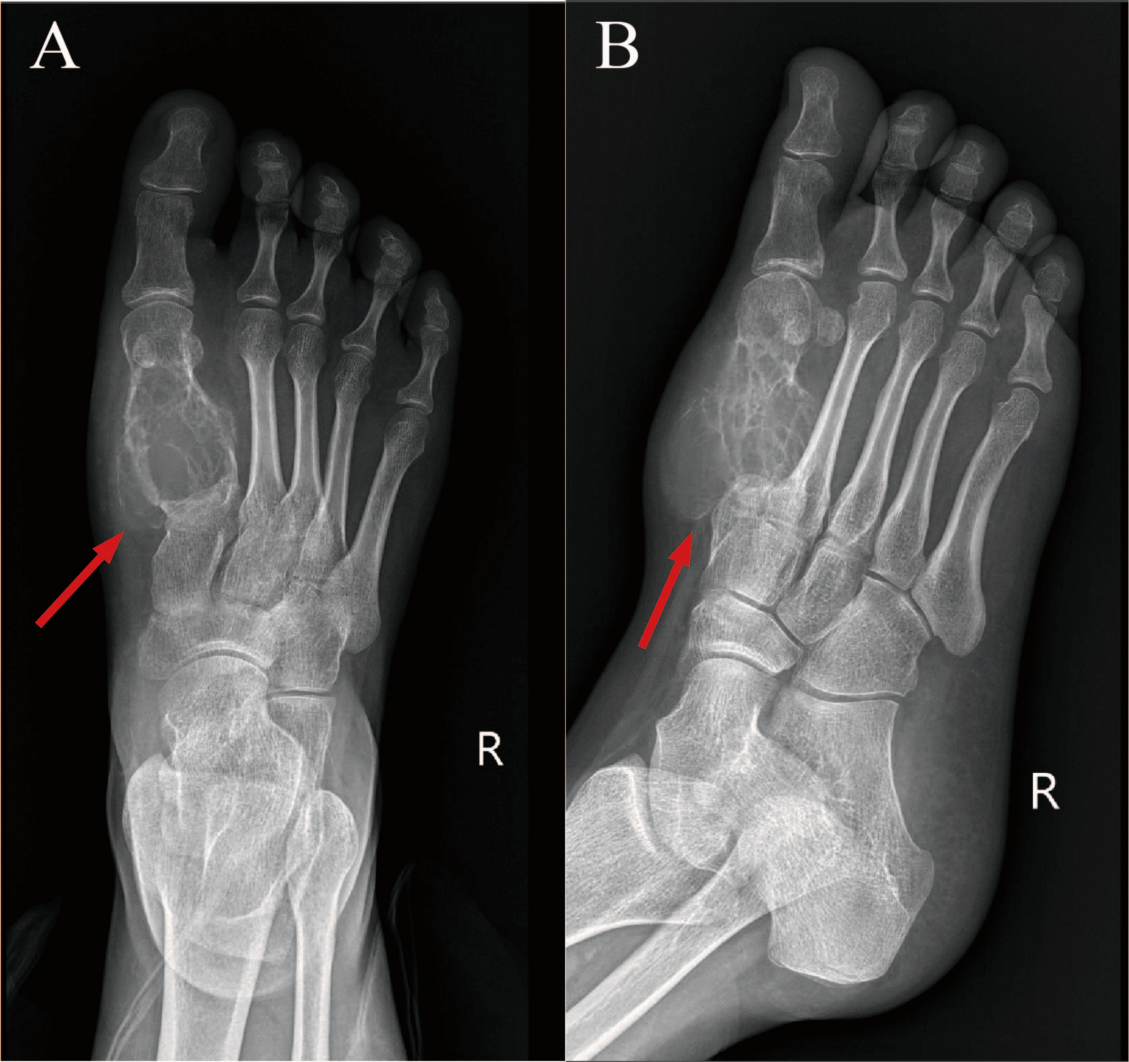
(A) AP and (B) lateral radiographs (A) AP and (B) lateral radiographs show an expansile and osteolytic bone destruction area in the right first metatarsal bone without an obvious periosteal reaction. The boundary with adjacent tissues remained clear, and there was swelling of the surrounding soft tissue.
